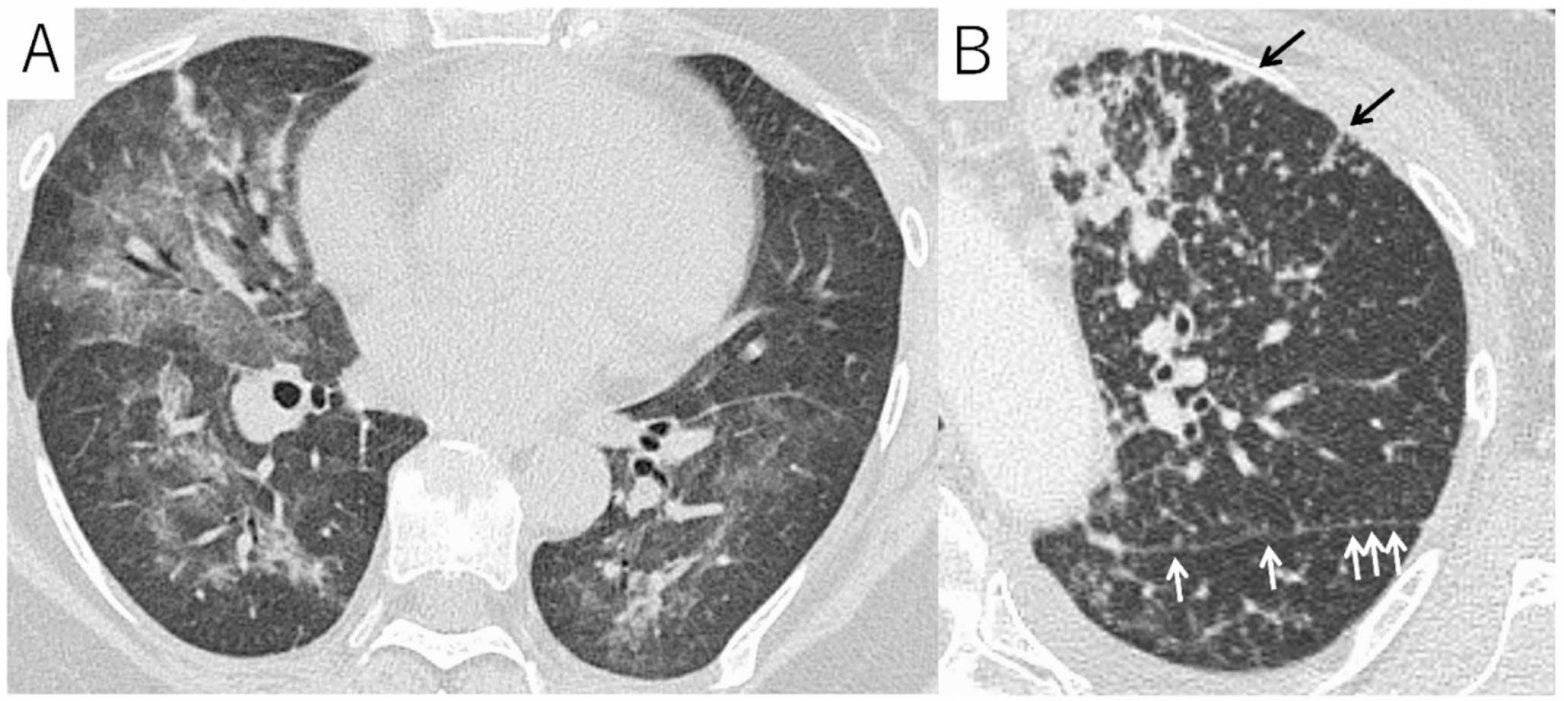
Differential diagnosis of drug-associated interstitial lung disease (DILD). A Pneumocystis pneumonia in a 56-year-old woman with breast cancer. A High-resolution CT (HRCT) shows bilateral extensive ground-glass opacities with peribronchovascular predominance. Although DILD was initially suspected, serum β-D glucan levels were elevated, and the patient was diagnosed with pneumocystis pneumonia. B Lymphangitic carcinomatosis in a 57 years-old woman with breast cancer. HRCT image shows multiple large nodules and micronodules. The micronodules extend along the interlobular septa and pleura, and beaded thickenings of the interlobular septa are also seen (arrows), suggesting lymphatic spread
